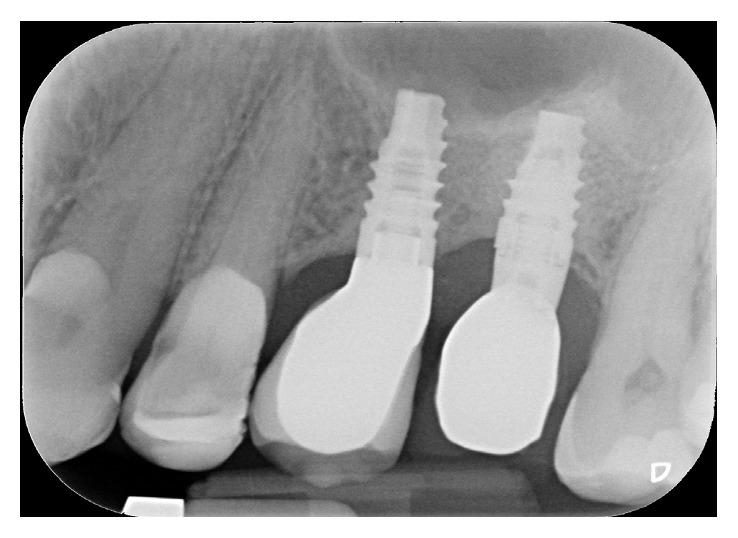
4 years' follow-up radiography. Sinus floor level is maintained on the top of both implants' apex and appears well corticalized. Crestal bone remodelling occurred around implant #26, not exceeding the smooth collar.
